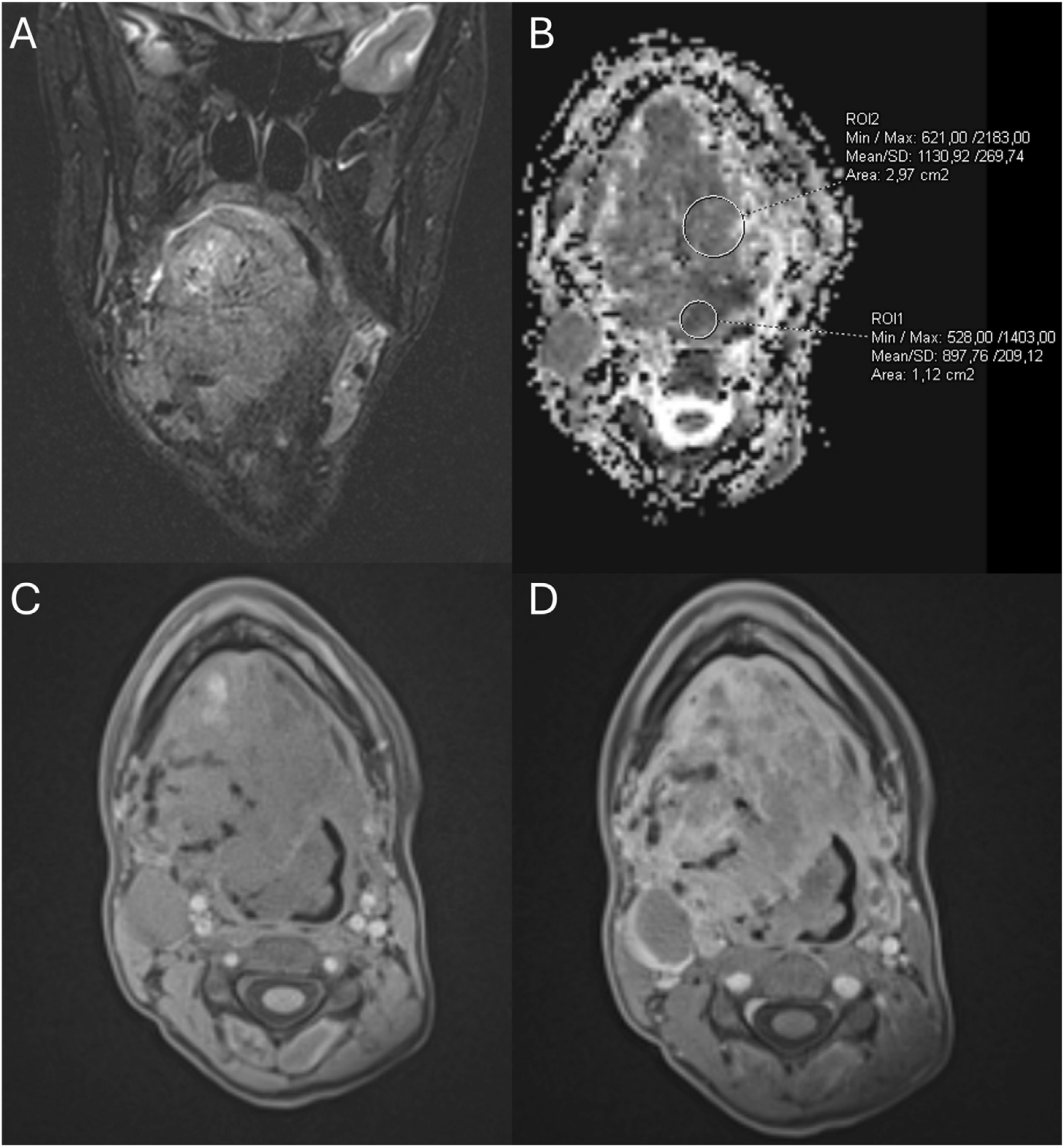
MRI examination at diagnosis of ASPS in December 2023. (A) Coronal T2-weighted MRI with fat saturation revealing a pathological mass in the tongue. (B) MRI-apparent diffusion coefficient (ADC) map displaying the pathological mass in the tongue. (C,D) Pre- and post-contrast T1-weighted gradient-echo MRI images showing vivid enhancement of the tongue mass and dilated surrounding vessels.
